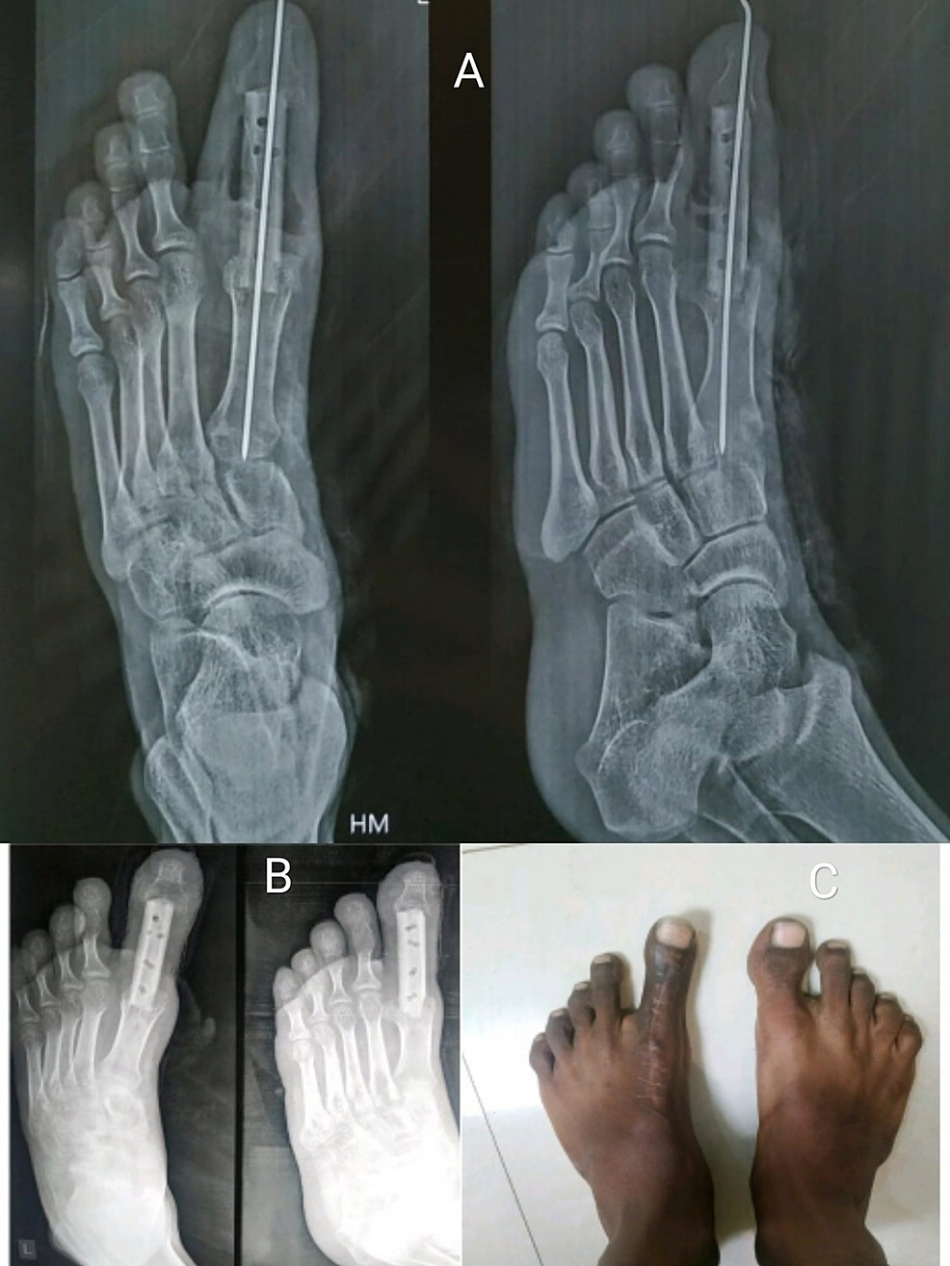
(A) Immediate postoperative X-ray. (B) Two-year postoperative follow-up X-ray. (C) Two-year follow-up clinical picture.
